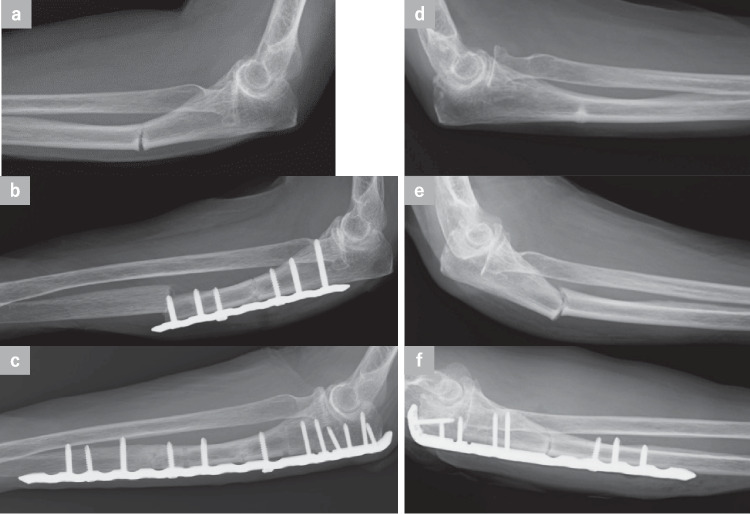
An 81-year-old woman (Patient No. 2) had been taking bisphosphonate (BP) preparations for more than 10 years for osteoporosis treatment. Pain developed in her left forearm after she threw an object. Radiographs revealed a transverse fracture in the proximal third of her left ulna and thickening of the dorsal cortex, indicating Stage II atypical ulnar fracture (AUF) (a). She was also diagnosed with a Stage I AUF on the right side (d). The right ulna fracture was displaced after 2 weeks and classified as an advanced Stage II AUF (e). She underwent open reduction-internal fixation using an anatomical locking plate (ALP) (VA-LCP olecranon plate; DePuy Synthes, Warsaw, IN) (f). Three weeks after surgery on the left forearm, a fracture was observed at the most distal point of the plate (b). Reoperation was performed by ALP (VA-LCP proximal ulnar plate; DePuy Synthes, Warsaw, IN) (c). Bony union was achieved on the left and right sides 8 and 6 months after the final surgery, respectively
